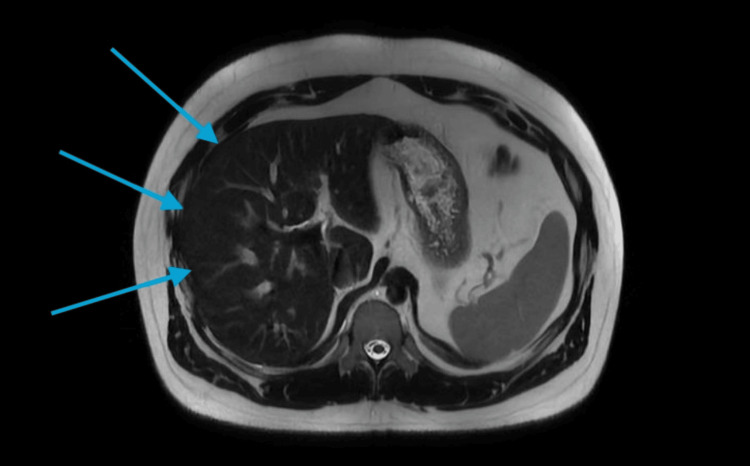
Magnetic resonance imaging showing diffuse signal loss on T2 imaging (arrows) throughout the liver which is present with evidence of signal increase on opposed gradient echo imaging indicating diffuse sign infiltration
